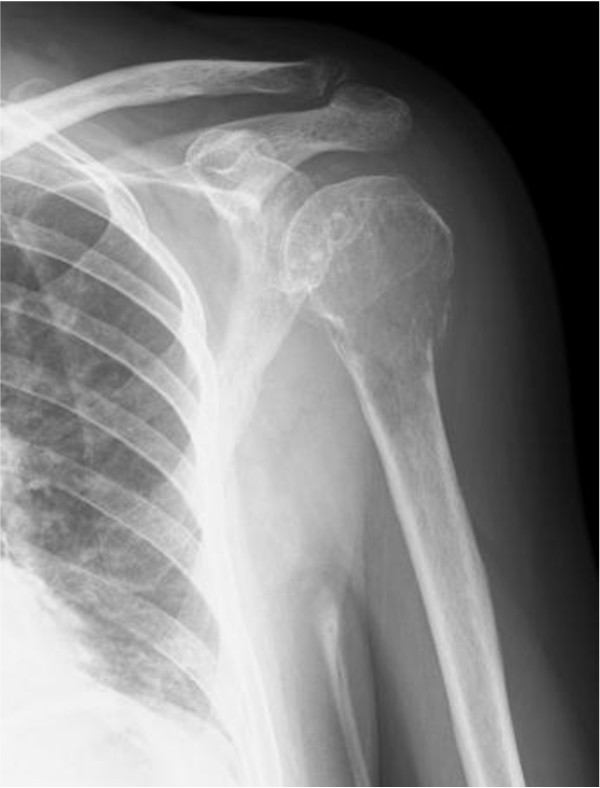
Anteroposterior radiograph of the left shoulder shows a pathological fracture with an osteolytic lesion.
